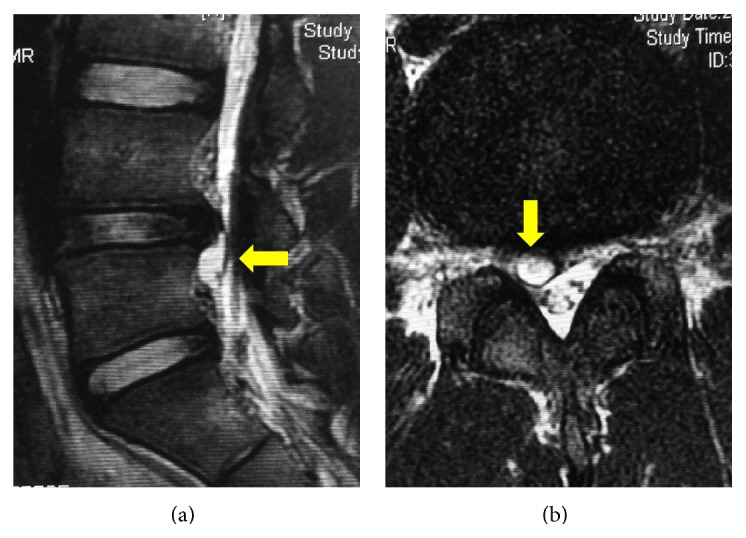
Preoperative magnetic resonance imaging at the 6-month follow-up: (a) sagittal T2-weighted and (b) axial T2-weighted images demonstrating the persistence of the cystic lesion (arrows).
